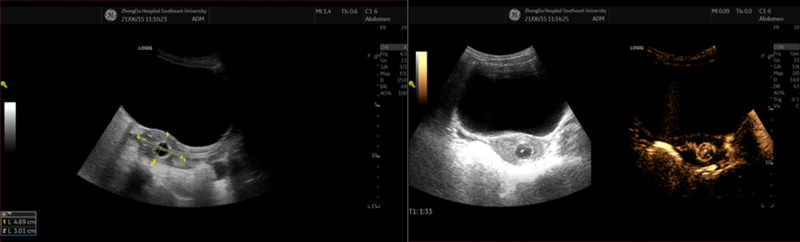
Postoperative ultrasonographic uterine microbubble angiography images (1 week after surgery).
